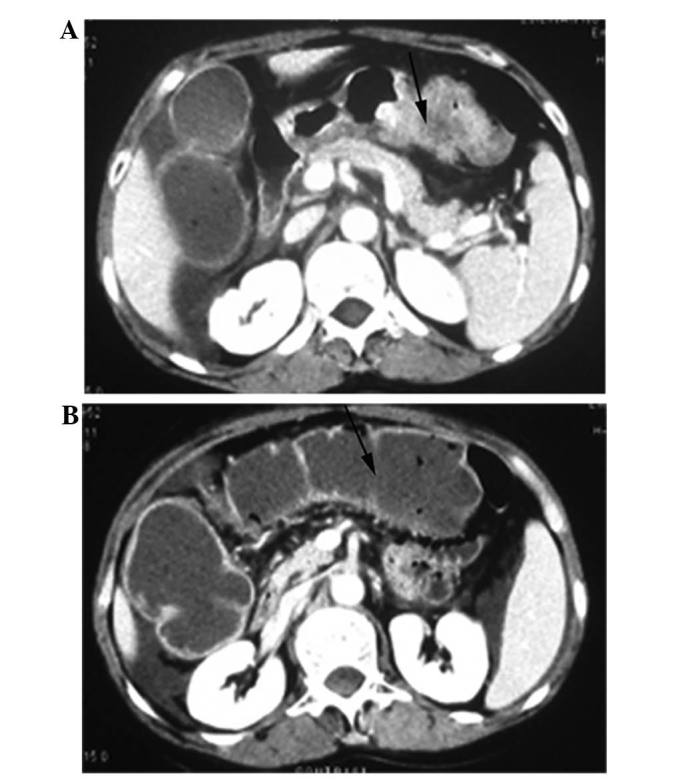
Computed tomography (CT) scan showing the gastric wall thickening and tumor infiltration into the (A) adjacent fat tissues and (B) colon, which were fluid-filled, indicative of a large bowel obstruction.
